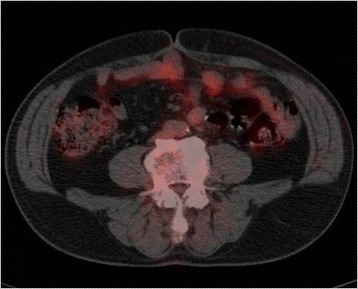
PET/CT imaging. PET/CT showing that the masses displayed increased FDG uptake
